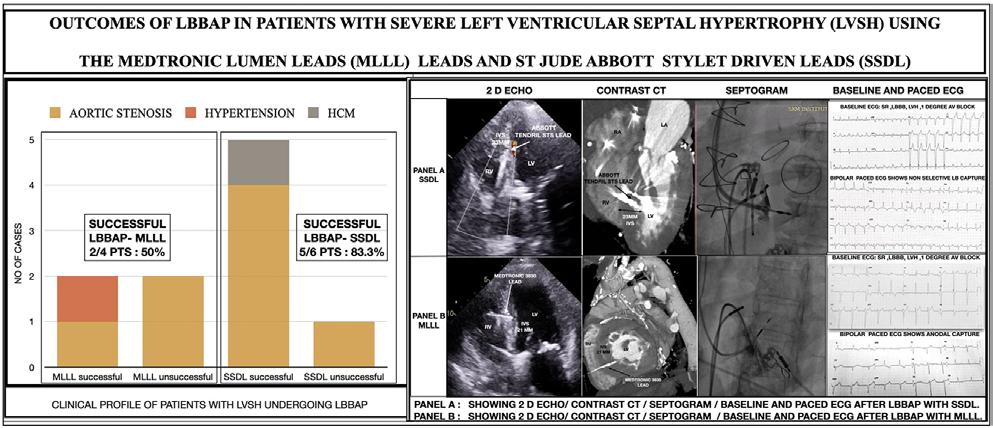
## NOVEL USE OF THE MEDTRONIC 3830 LEAD AS A BIOPTOME IN A CASE OF SUSPECTED INFILTRATIVE CARDIOMYOPATHY
